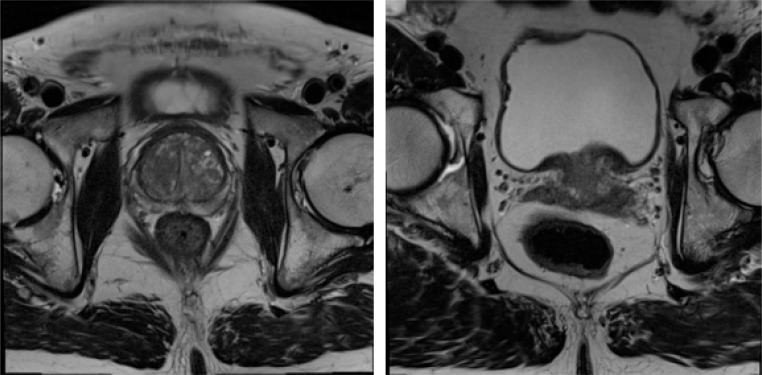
Transverse plane images of prostate from the dataset (the left one is a normal prostate’s MRI image, and the right one is an MRI image from a prostate cancer patient).
